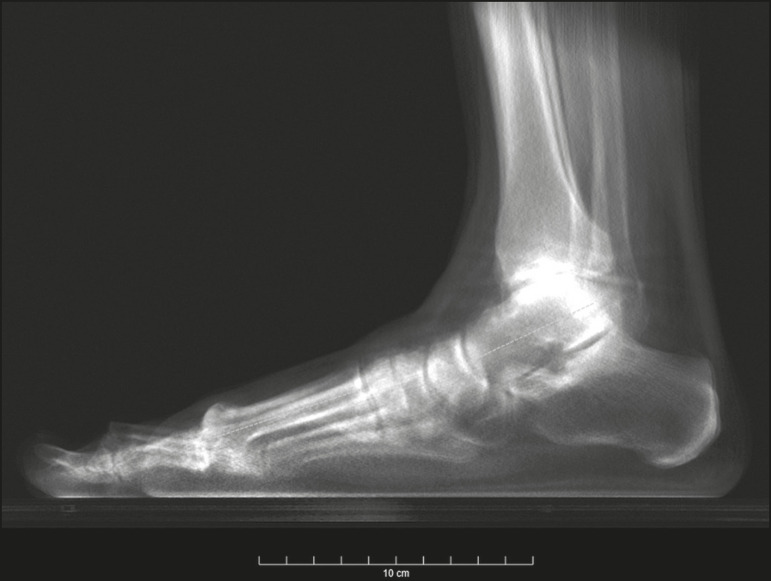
Lateral-view weight-bearing digital reconstructed radiograph of the ankle. Note the superposition and misalignment of the structures, leading to poor definition of the contours.
